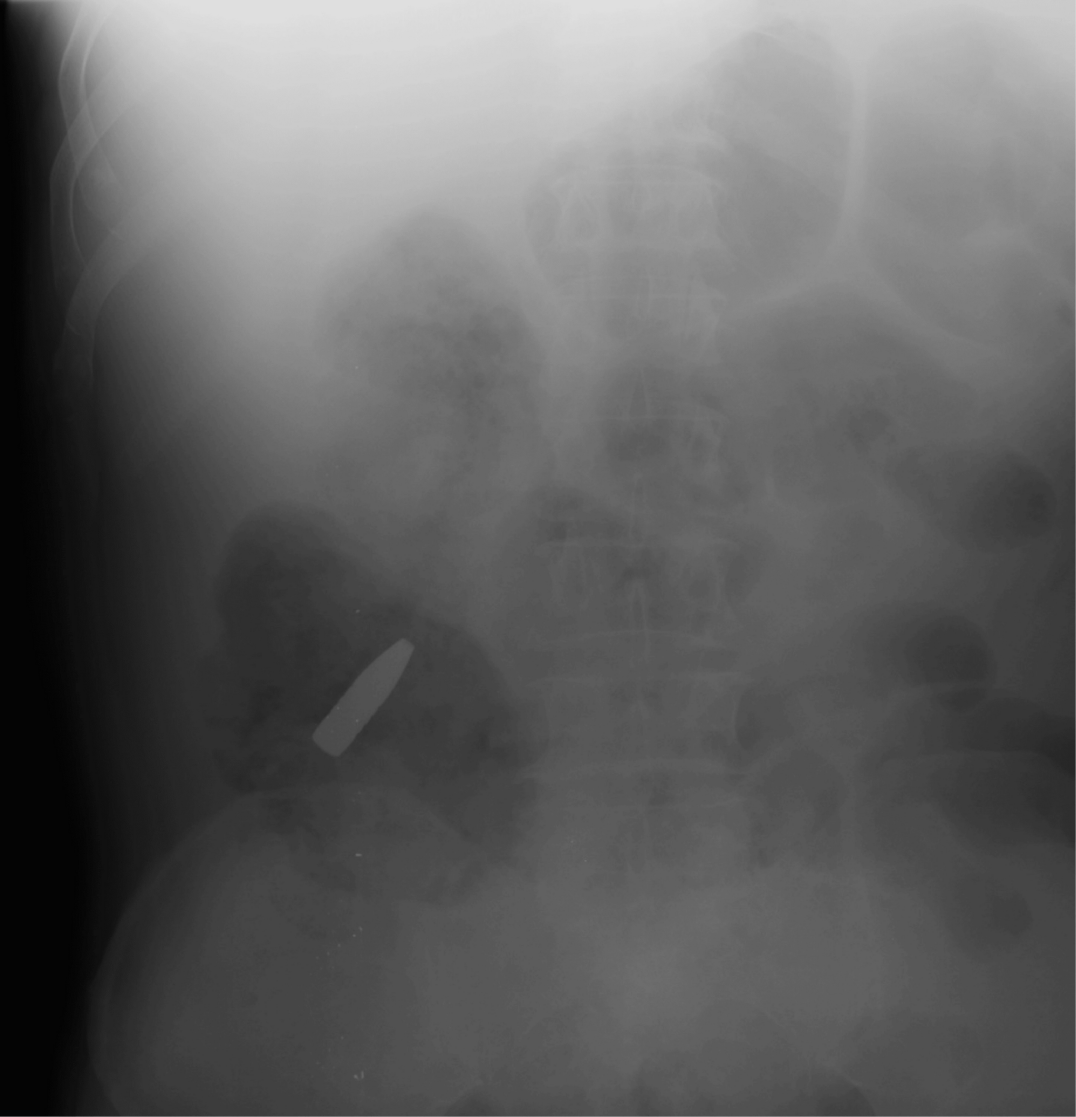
AP (anteroposterior) X-ray examination preceding the autopsy indicating the position of the bullet
